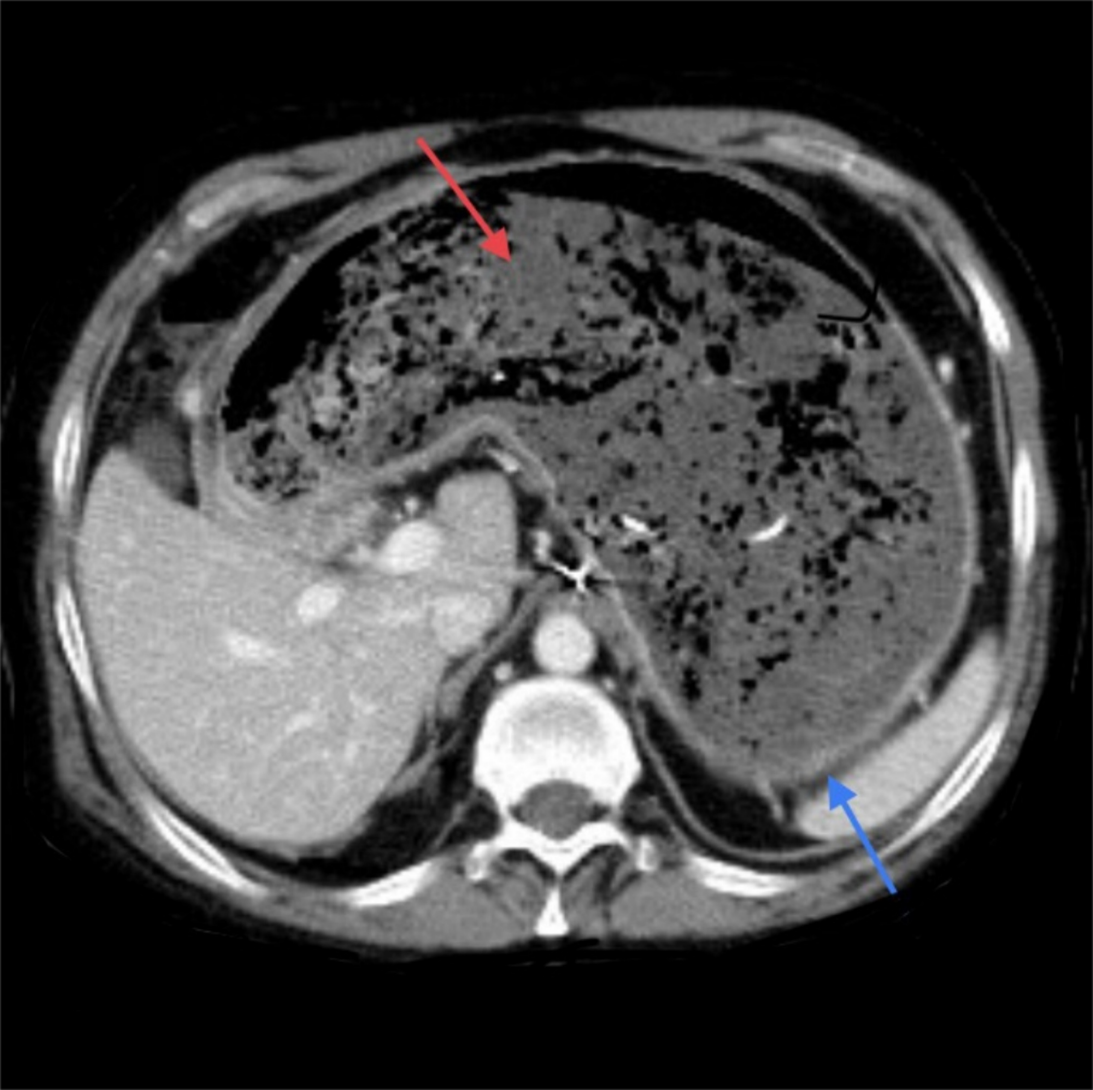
Computed tomography (CT) scan abdomen. Stomach (blue arrow). None enhancing mass (red arrow).
